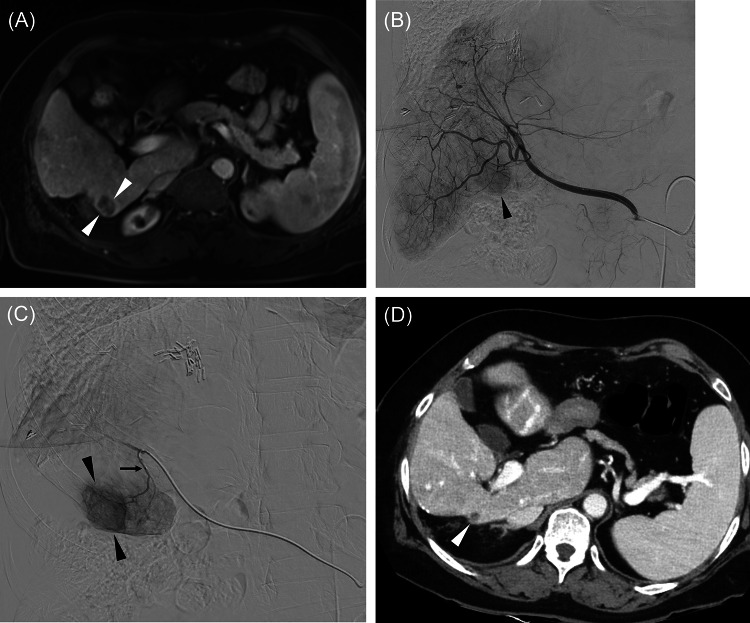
A 64-year-old woman with 2 cm sized single metastasis from colon cancer. She underwent low anterior resection and liver tumorectomy 11 years ago, followed by first-line chemotherapy. Six years ago, she experienced a recurrence with liver metastasis, for which tumorectomy was performed and second-line chemotherapy was administered. A Axial MR image of portal venous phase shows a 2 cm nodular tumor (arrowheads). B Right hepatic angiogram shows a tumor blush (arrowhead). C Selective angiogram of subsegmental branch shows small tumor blush (arrowheads). Resin microspheres of 0.4 GBq were infused at the subsegmental branch. Note a microcatheter tip (arrow). D CT scan 6 months after radioembolization shows partial response of the tumor (arrowhead)
